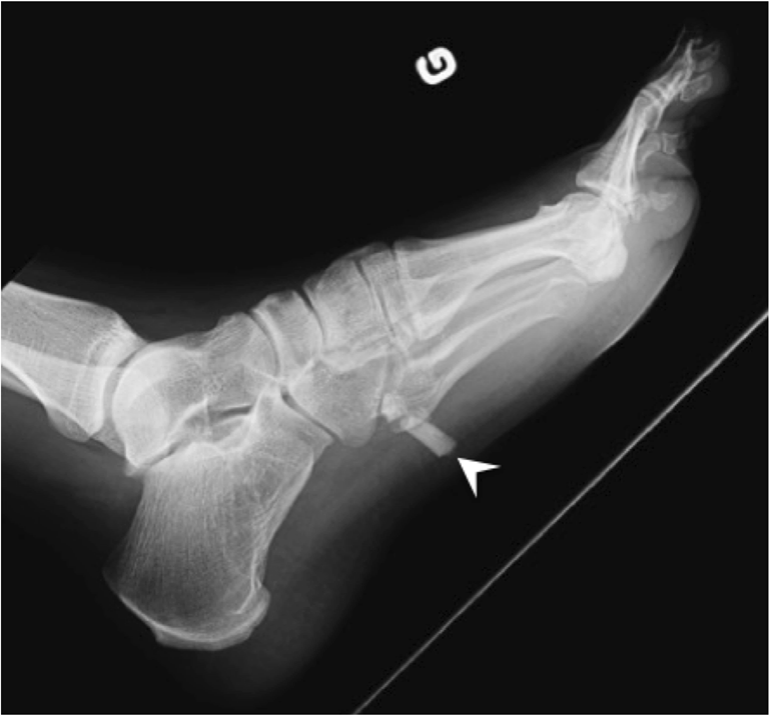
Foreign bodies. Lateral radiograph of the foot shows glass fragments (arrowhead) in the plantar soft tissues, with sharp edges and a rectangular shape
